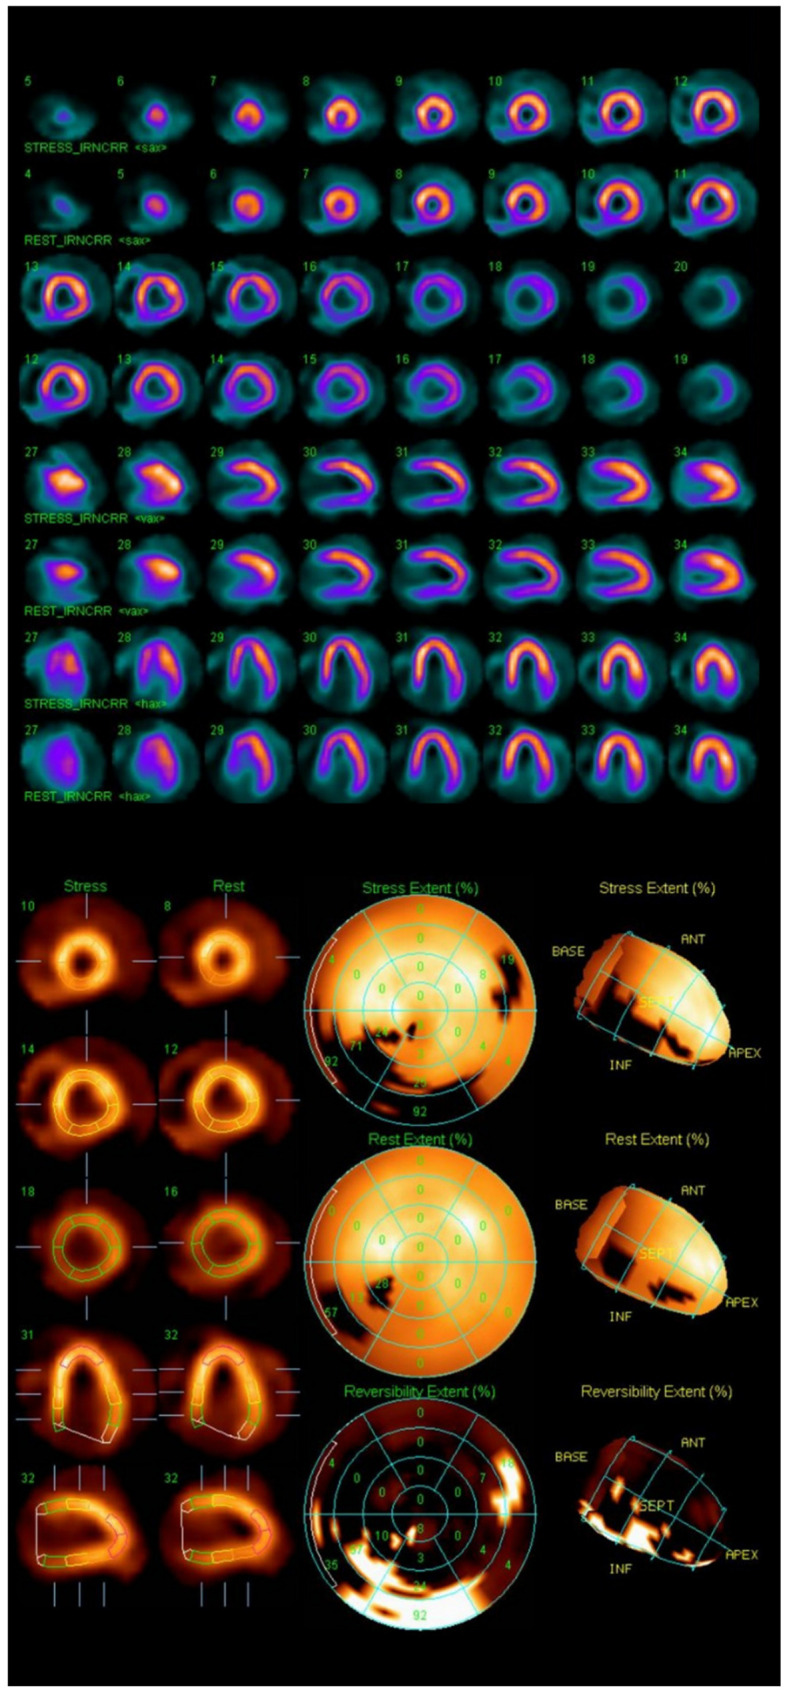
A 65-year-old male patient with anomalous origin of the right coronary artery from the left/posterior coronary sinus of Valsalva associated with an anomalous/malignant intraarterial proximal coronary course of the right coronary artery (between the pulmonary artery anteriorly and the aorta posteriorly; probable intramural nature of the paraostial coronary segment). Myocardial perfusion SPECT with technetium-99m tetrofosmin performed after pharmacologic stress with regadenoson shows a moderate myocardial hypoperfusion in the infero-septal area, almost completely reversible in the rest images, consistent with stress-induced ischemia in the right coronary artery territory. Left ventricular dilation with mild reduction in contractile function.
